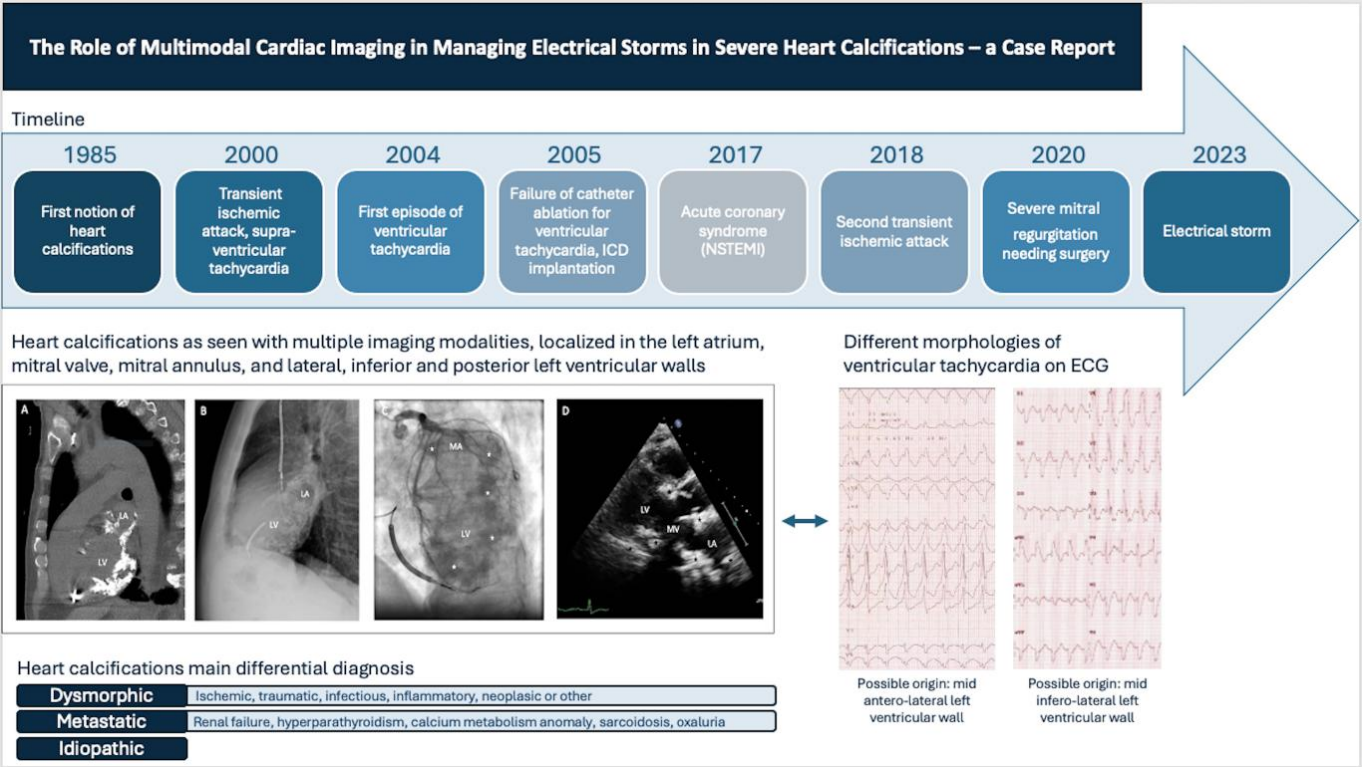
(*A*) Non-contrast computed tomography (CT) showing extensive amorphous confluent calcifications in the left ventricular (LV) wall, sparing the septum, and extending to the mitral-aortic annulus and the LA. (*B*) Chest radiography showing calcifications in the LV wall, extending to the mitral-aortic annulus and the LA. (*C*) Calcifications (marked with white stars) in the LV myocardium and the mitral annulus (MA) as seen on fluoroscopy during the coronary angiography. (*D*) Transthoracic echocardiographic parasternal long-axis view showing the extensive calcifications (marked with black stars) of the mitral valve (MV), extending to the mitral-aortic annulus, with intramyocardial calcification of the posterobasal and basal anteroseptal LV walls, and significant calcification of the LA. ICD indicates implantable cardioverter-defibrillator and NSTEMI myocardial infarction without ST elevation.
